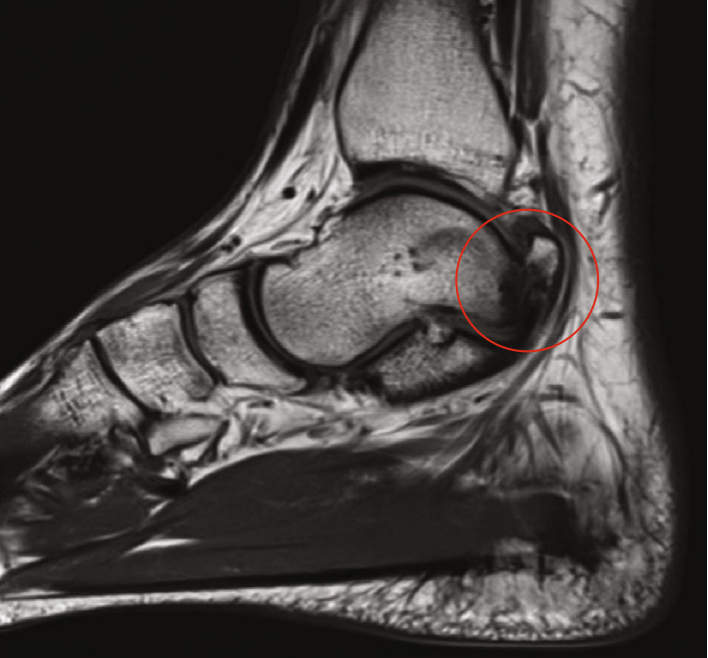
Sagittal slice of T2 MRI of right ankle showing the posteromedial talus fracture fragment and associated oedema.
